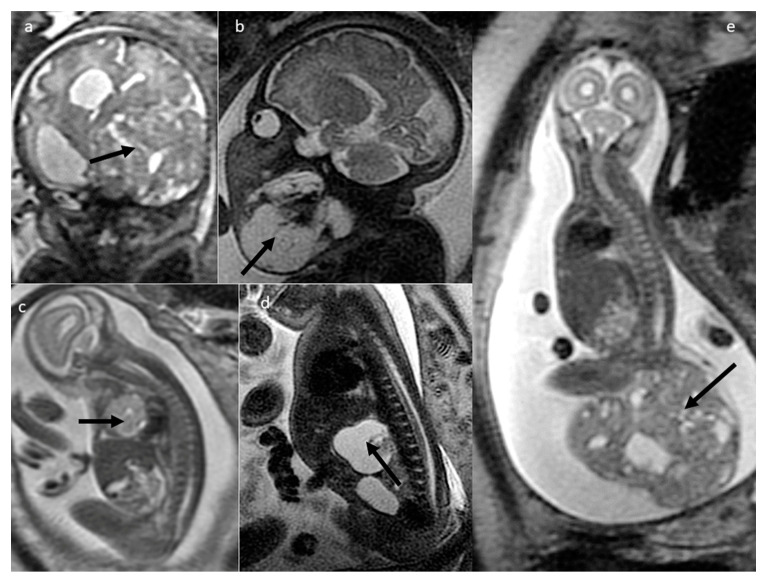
Different locations of teratomas (arrows) on fetal MRI (SSFSE/T2W and FIESTA images). (a) Intracranial; (b) facial; (c) anterior mediastinal; (d) abdominal; (e) sacrococcygeal (SCT type II).
